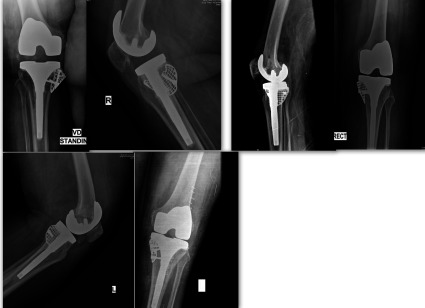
Post-operative X-rays with incorporation of impacted bone graft and well-aligned knee prosthesis.
